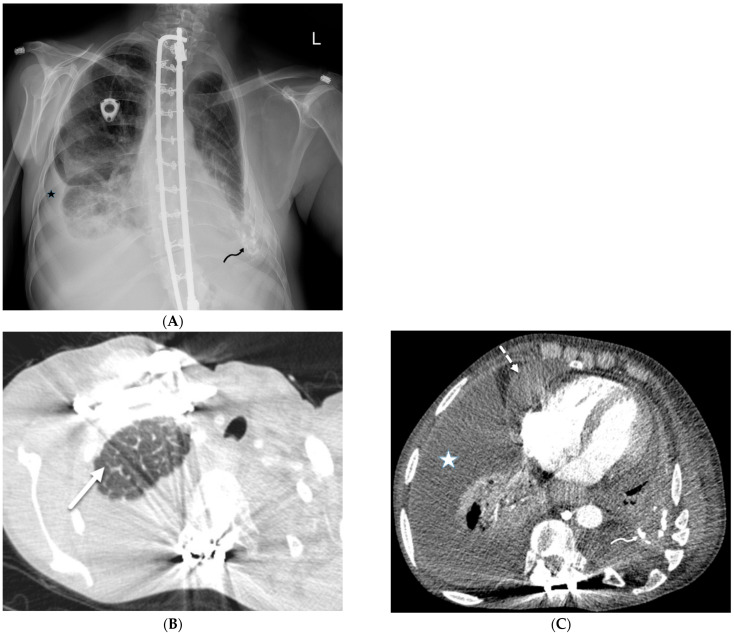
A 53-year-old female diagnosed with congenital pulmonary lymphangiectasia at birth. A frontal chest radiograph (A) and axial chest CT images (B,C) demonstrate smooth interlobular septal thickening (solid white arrow), bilateral pleural effusions (star), and pericardial effusion (dashed white arrow). High attenuation foci in the left pleural space (curved arrows) are related to prior pleurodesis.
